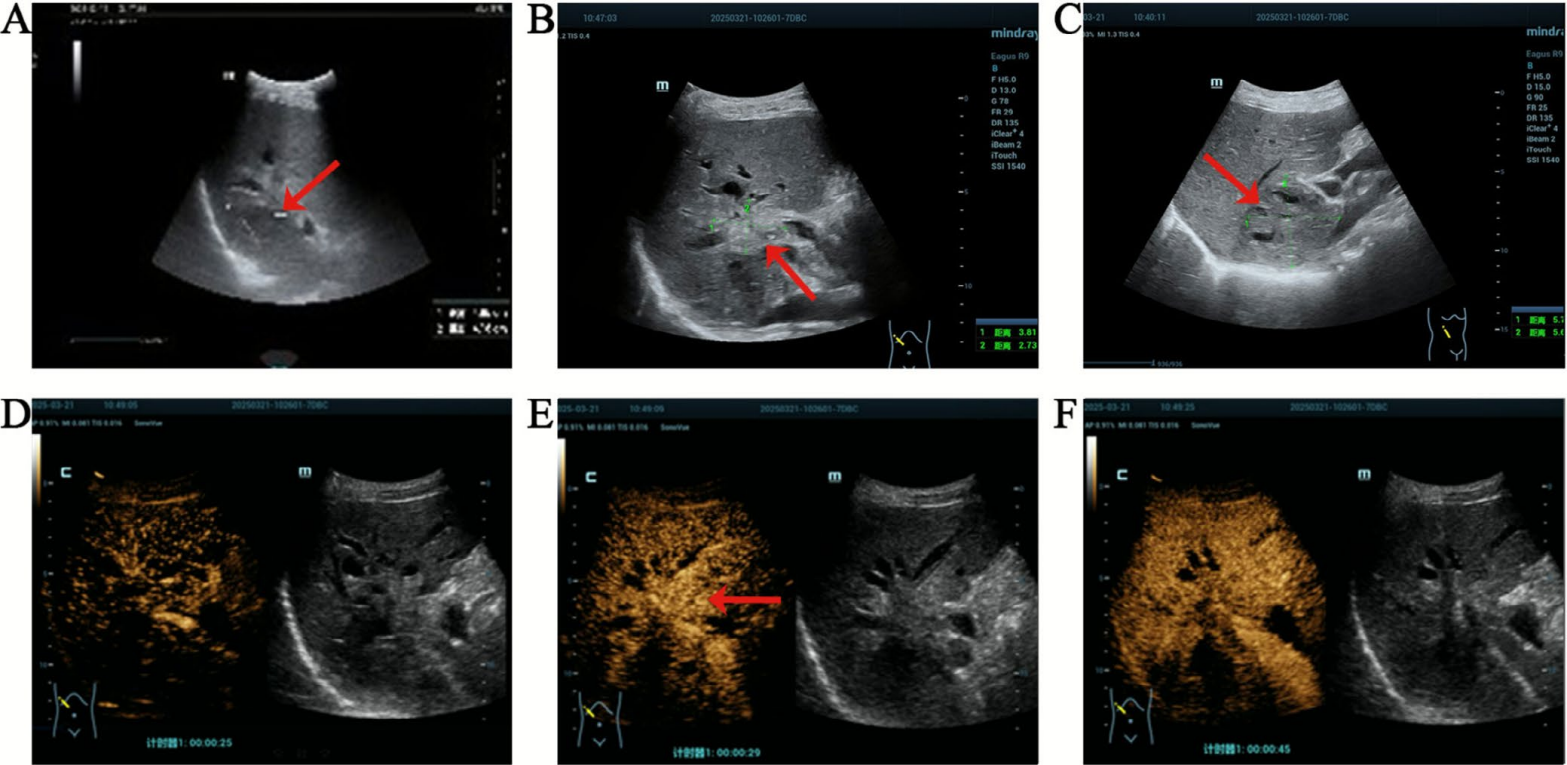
Ultrasound and contrast‐enhanced ultrasound (CEUS). (A) Routine ultrasound on March 16 showed patchy hypoechoic areas in the right lobe of the liver; (B) Ultrasound reexamination on March 21 showed a slightly hyperechoic mass at the hepatic hilum. (C) In the right sector (segments V to VIII) of the liver, there was a solid predominantly cystic and solid mixed echo area near the dome of the diaphragm. (D) CEUS, liver parenchyma imaging began at 22 s; (E) The enhancement peaked at 29 s; (F) Overall hypoenhancement of the mass for 45 s.
